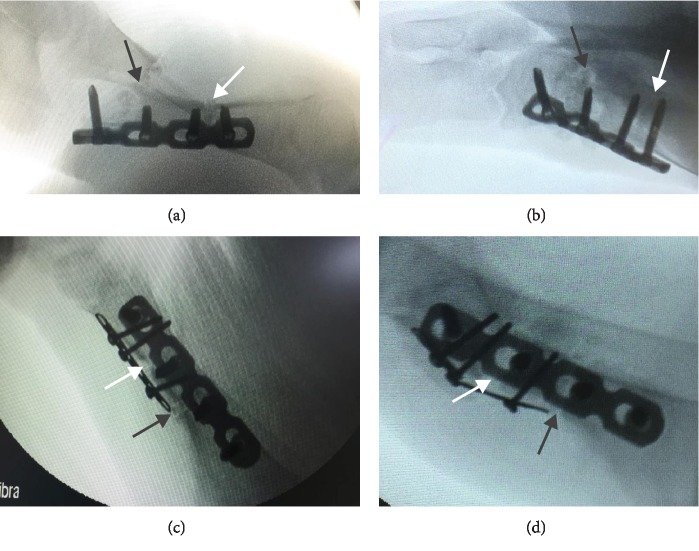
Models of defects C, A1, and A2 and radiography. Time-dependent radiological changes in the defect, 4 and 8 weeks after reconstruction. (a) 4 weeks after reconstruction, the *Tibia 1* showing failed defect C (black arrow) and callus formation between the cortex gap of defect A1 (white arrow). (b) 8 weeks after reconstruction, much more callus connecting two ends of defect A1. (c) 4 weeks after reconstruction, the *Tibia 2* showing more callus formation in control defect C (black arrow) than in treatment defect A2 (white arrow). (d) 8 weeks after reconstruction, broken plate happens, whereas more callus in defect C.
